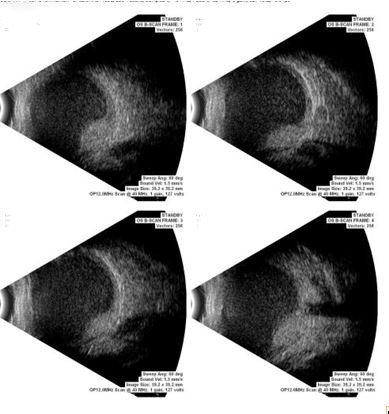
B-scan ultrasonography image showing heterogenous mass in the posterior segment, hypoechoic anteriorly and hyperechoic posteriorly
